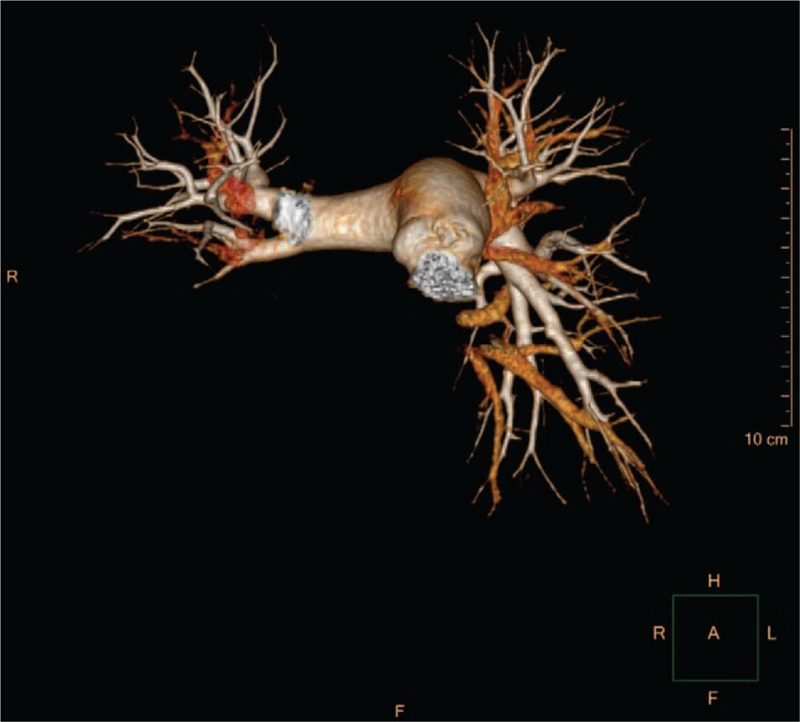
CTA of pulmonary artery. CTA revealed pulmonary artery of right lower lobe was not visualized. CTA = computed tomography angiography.
